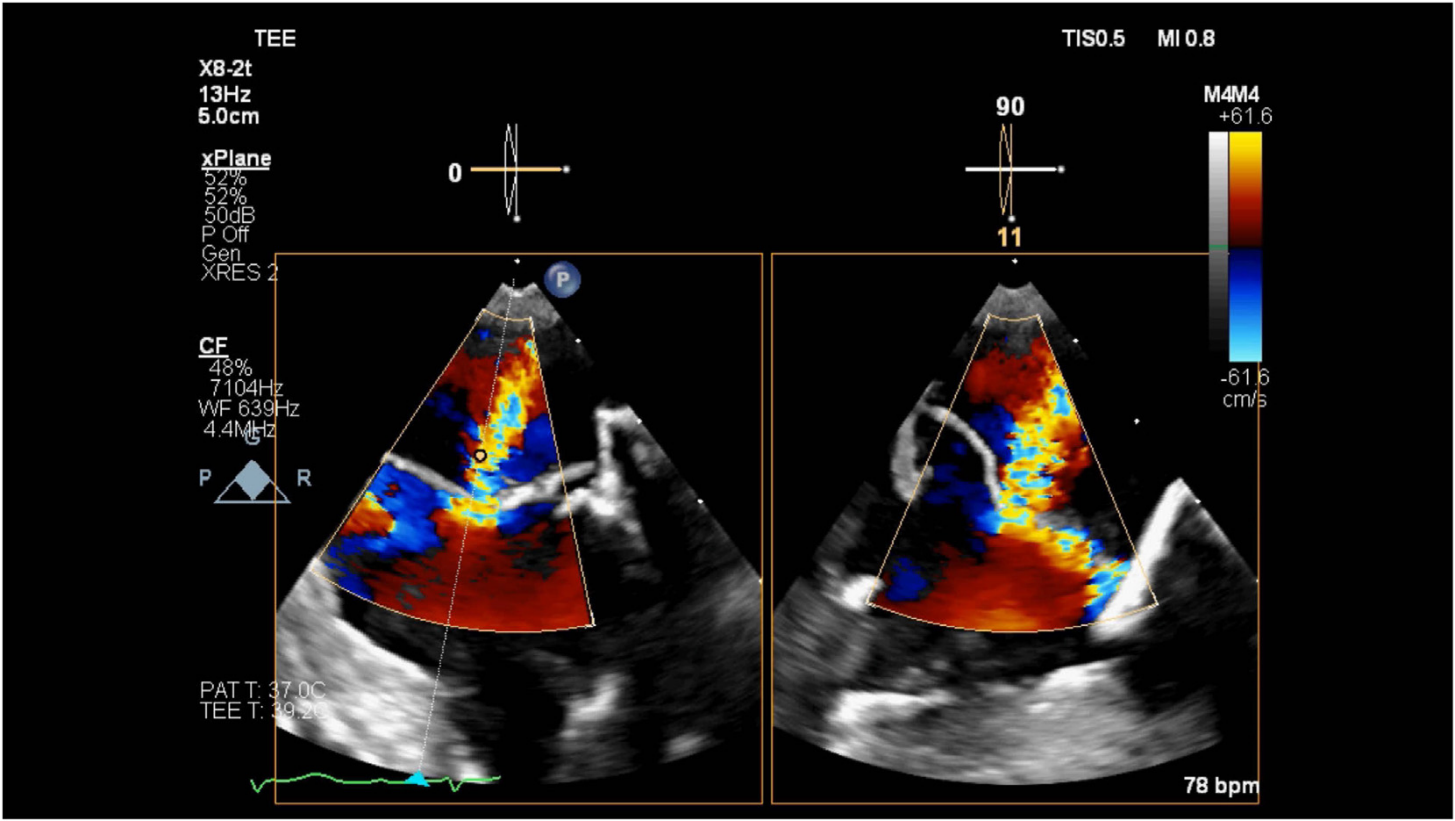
Two-dimensional TEE, midesophageal 4- (*left*) and 2- (*right*) chamber views (foreshortened) with color-flow Doppler, systolic phase, demonstrates severe valvular MR following bioprosthetic MVR.
